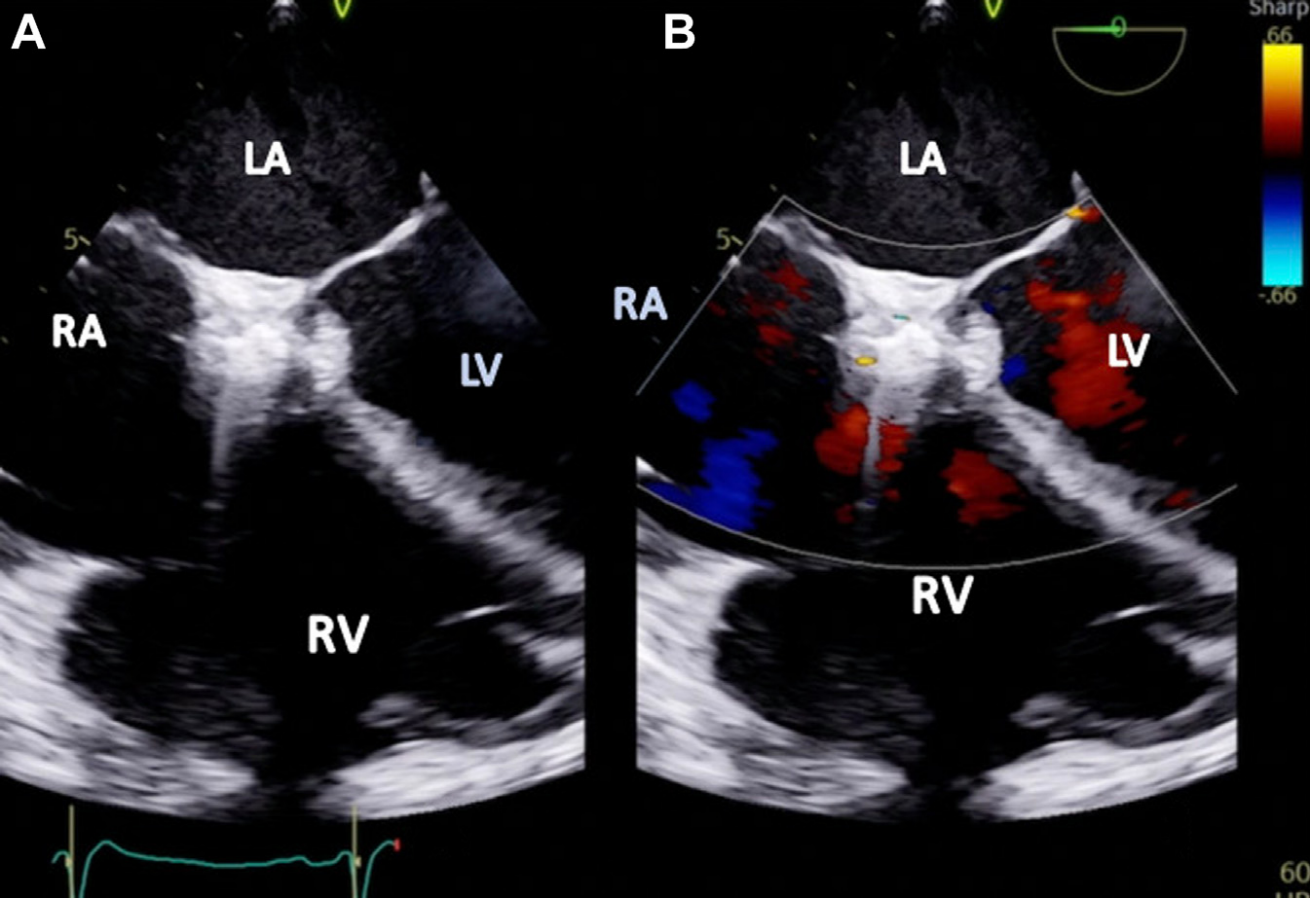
Transesophageal Echocardiographic Images Showing Gerbode Defect Closure (A) Without Doppler. (B) With color Doppler. These images did not reveal any flow between the left ventricle (LV) and the right atrium (RA), and they show successful closure of the Gerbode defect. Abbreviations as in Figures 2 and 3.
